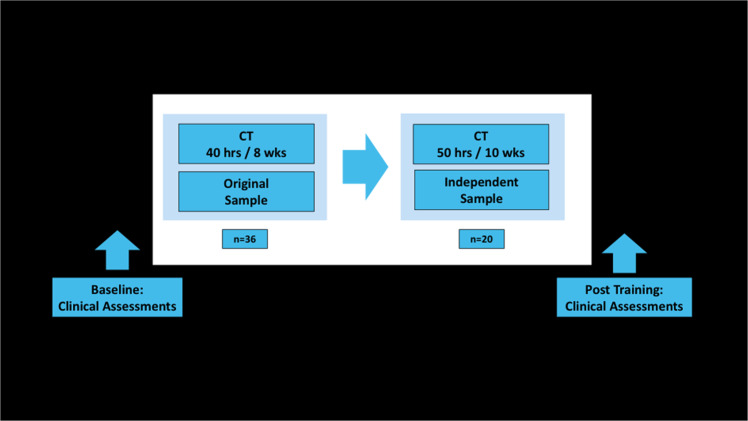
Training design of the original and independent validation samples. The machine learning support vector model reliably predicted GAF ≥ 45 vs. GAF < 45 in SCZ participants in response to auditory-based cognitive training (CT) at the single-subject level in both samples.
